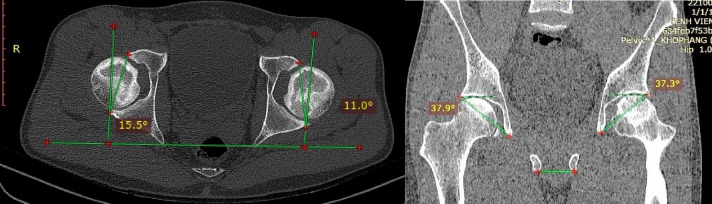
Preoperative CT-MPR-based measurement of acetabular anteversion angle and acetabular inclination angle^4^.
